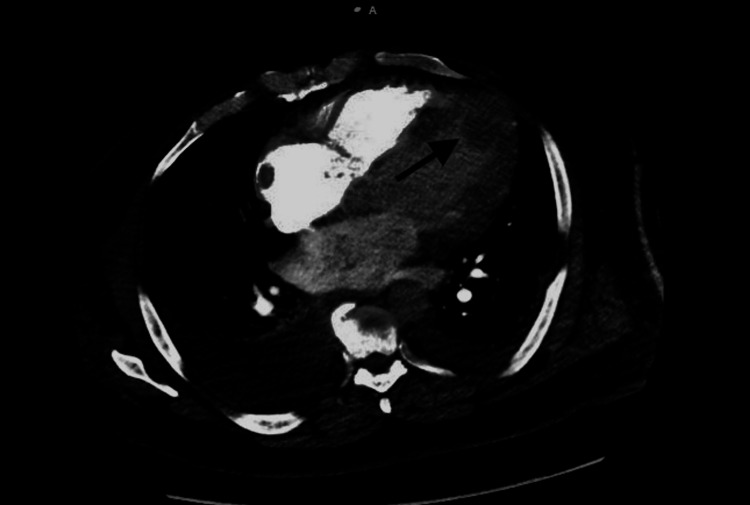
CT angiography of the chest showing a mass in the apical region
